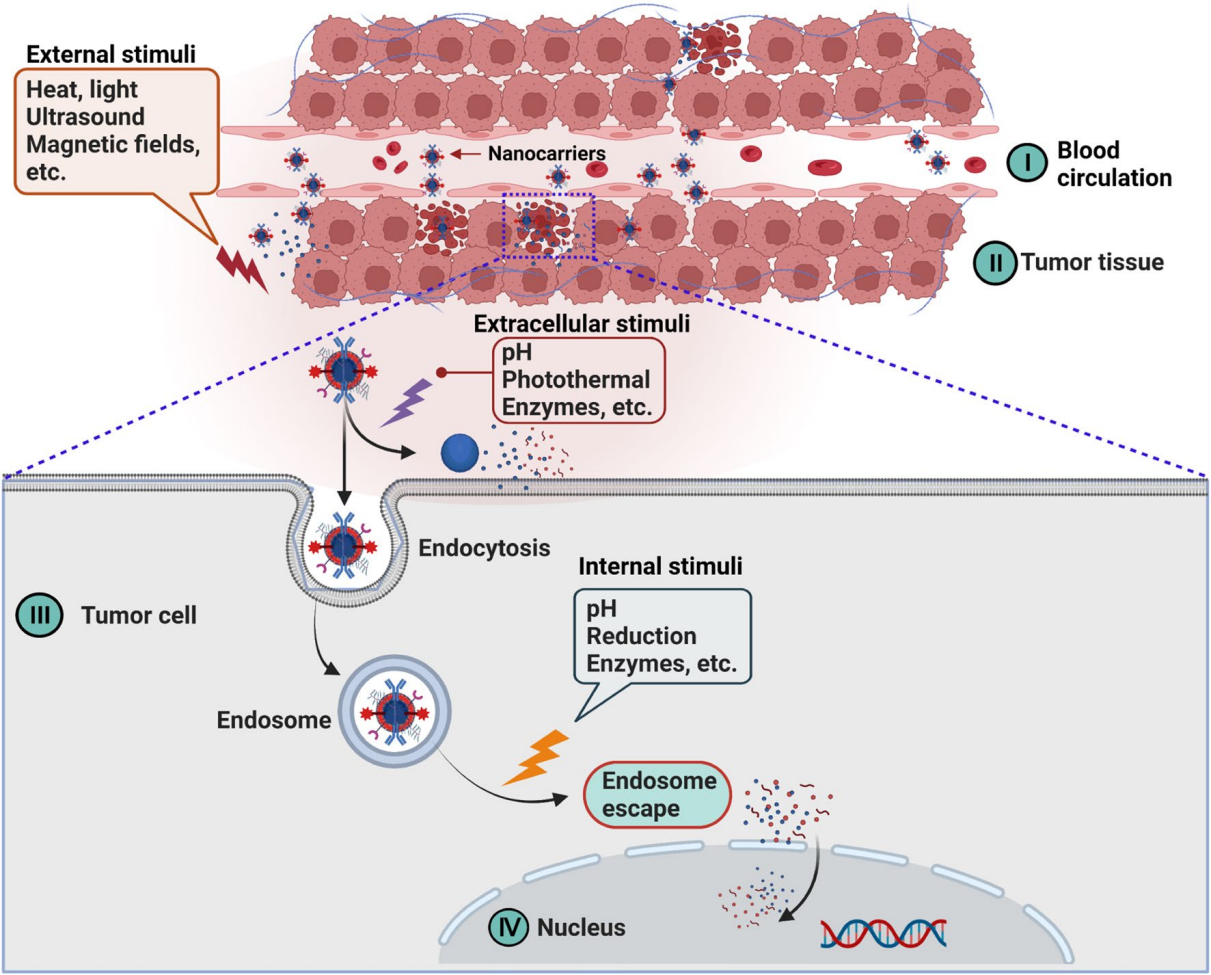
Nanocarriers penetrate from blood circulation (I) into the tumor nucleus (IV) and are responsive to different stimuli. (I − II) Drug delivery systems based on nanocarriers can enhance drug penetration and retention into the tumor tissues via the endothelial gap. Externally stimuli (e.g., heat, light, ultrasound, magnetic fields, etc.) or extracellular stimuli (e.g., acidic TME, thermal radiation, enzymes, etc.) can cause the nanocarriers to release their cargos in the vicinity of the target tissue. (III − IV) Intracellular nanocarriers escape from the endosomes and release their drugs in response to internal stimuli (e.g., pH, reduction, enzyme, etc.) and specifically bind to the target to exert anticancer effects. The figure has been created using Biorender
